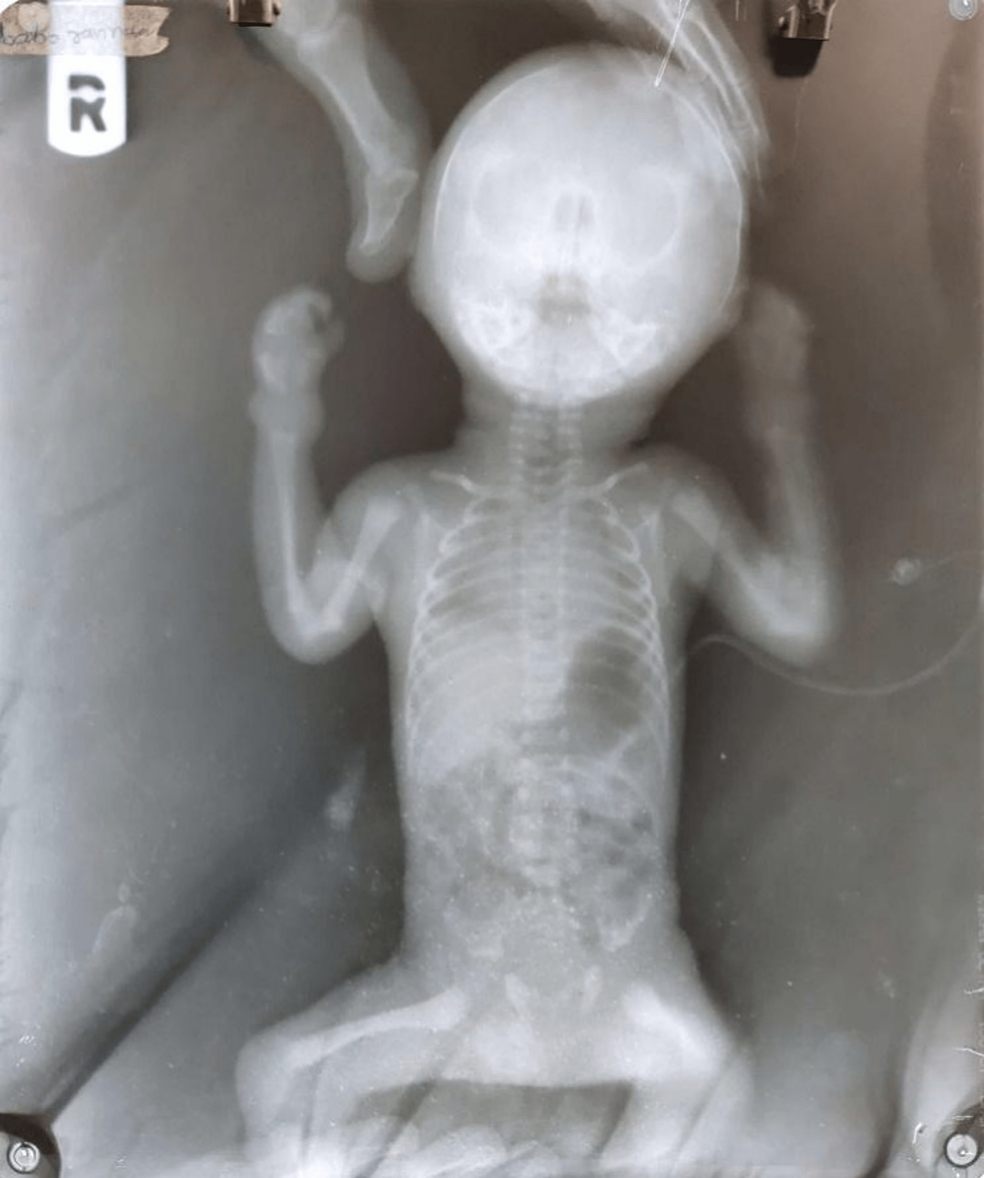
A radiographic X-ray of the baby Subluxation of joints was present.
